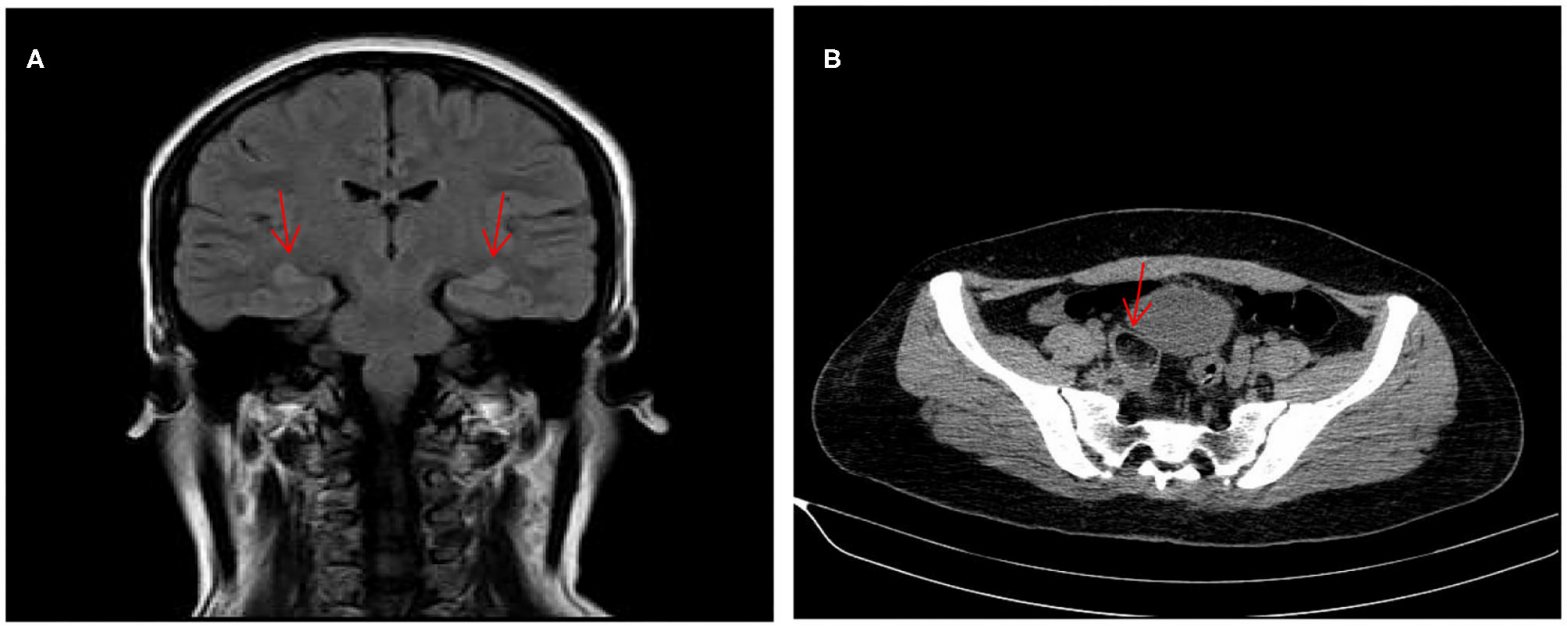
MRI of the brain revealed subtle increased signal intensity in bilateral hippocampal on FLAIR sequences (A). CT scan of the abdomen indicated a space-occupying lesion in right-side ovarian, later pathologically identified as mature ovarian cystic teratoma (B).
